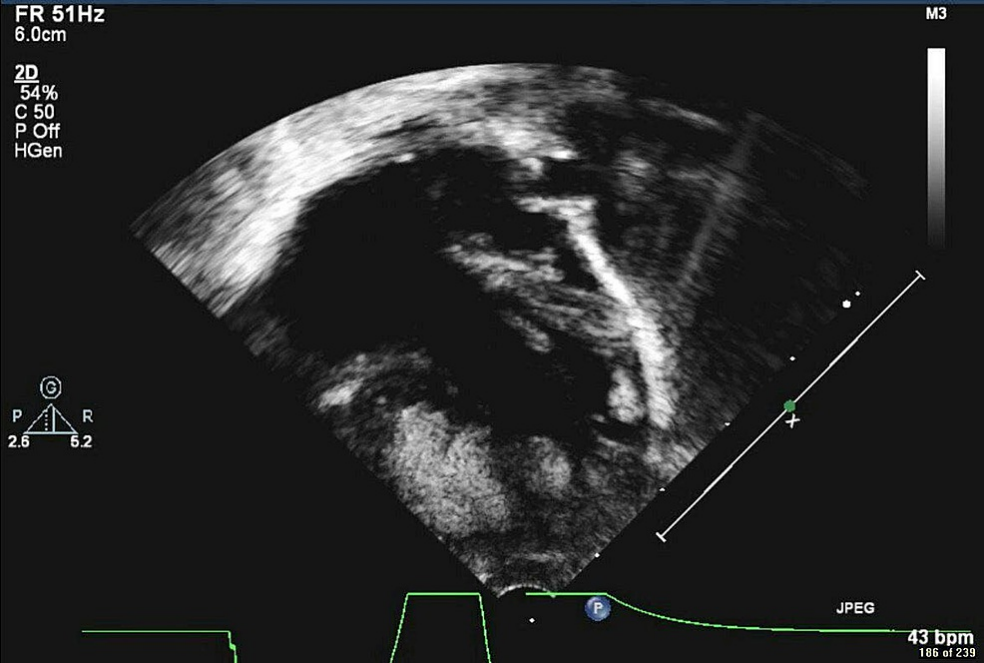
Echocardiography shows severe hypoplastic left ventricle
